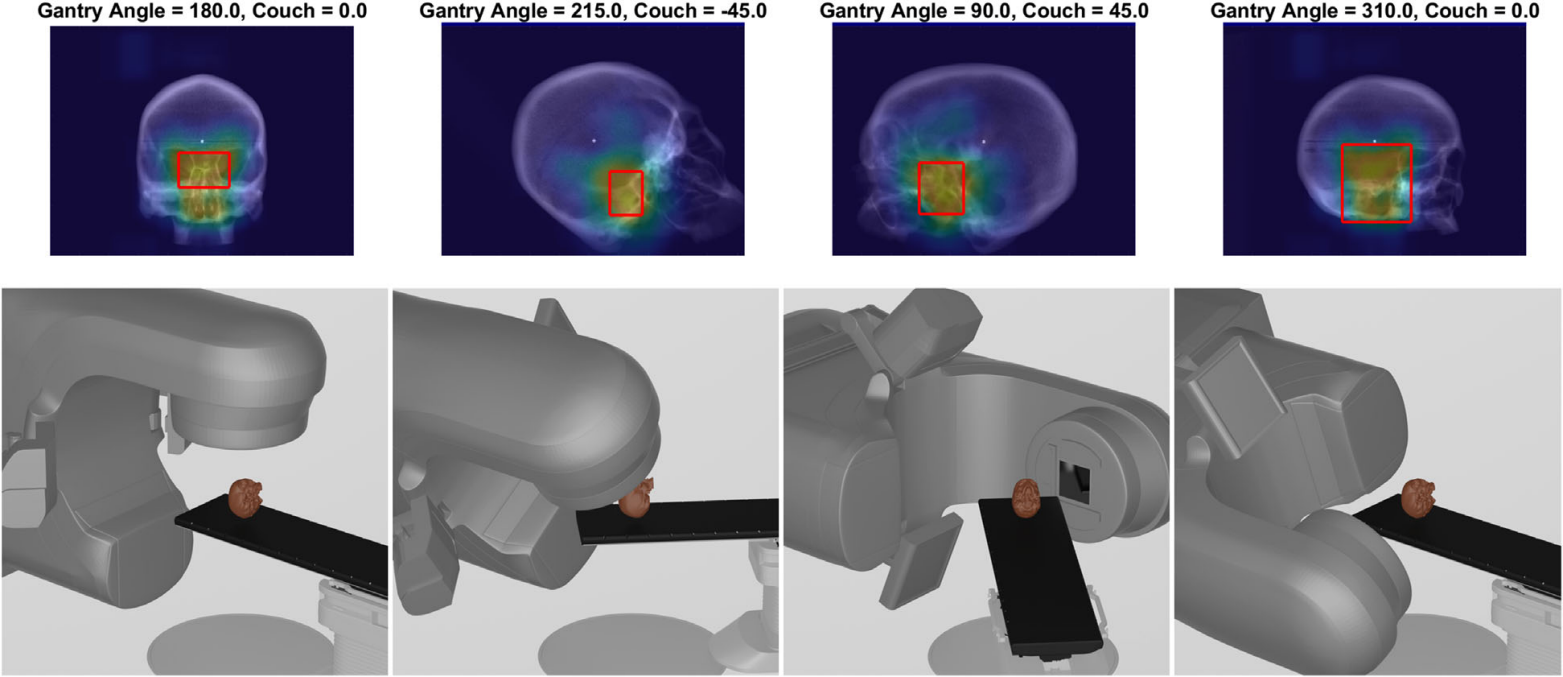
Examples of the top 10% of apertures that minimized registrations errors for four different beam's eye view (BEV) depicted as a color wash overlaid onto a digitally reconstructed radiograph (DRR). Bright colors highlight the anatomical regions shared by the proportion of the top 10% of apertures. The red box articulates the 80% threshold level for the creation of an imaging aperture for the respective BEV. The accompanying couch and gantry positions are depicted in the row below the BEV.
